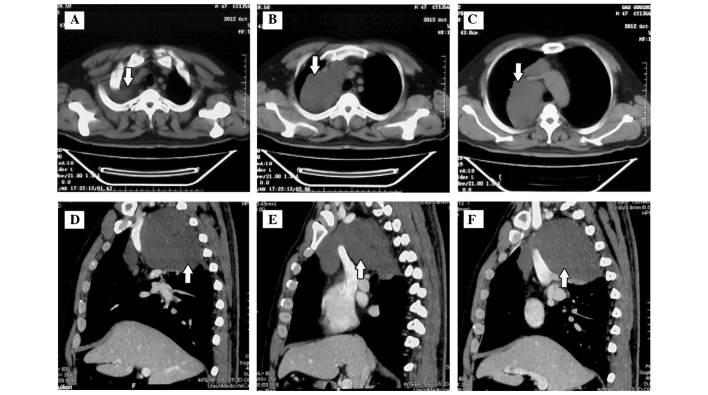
Thoracic computed tomography images of the mediastinal tumor. (A-C) Thoracic cross-sectional imaging showing the different layers of the tumor. The lesion is indicated by downward-pointing arrows. (D-F) Thoracic vertical section imaging showing the layers of the tumor, indicated by upward-pointing arrows.
